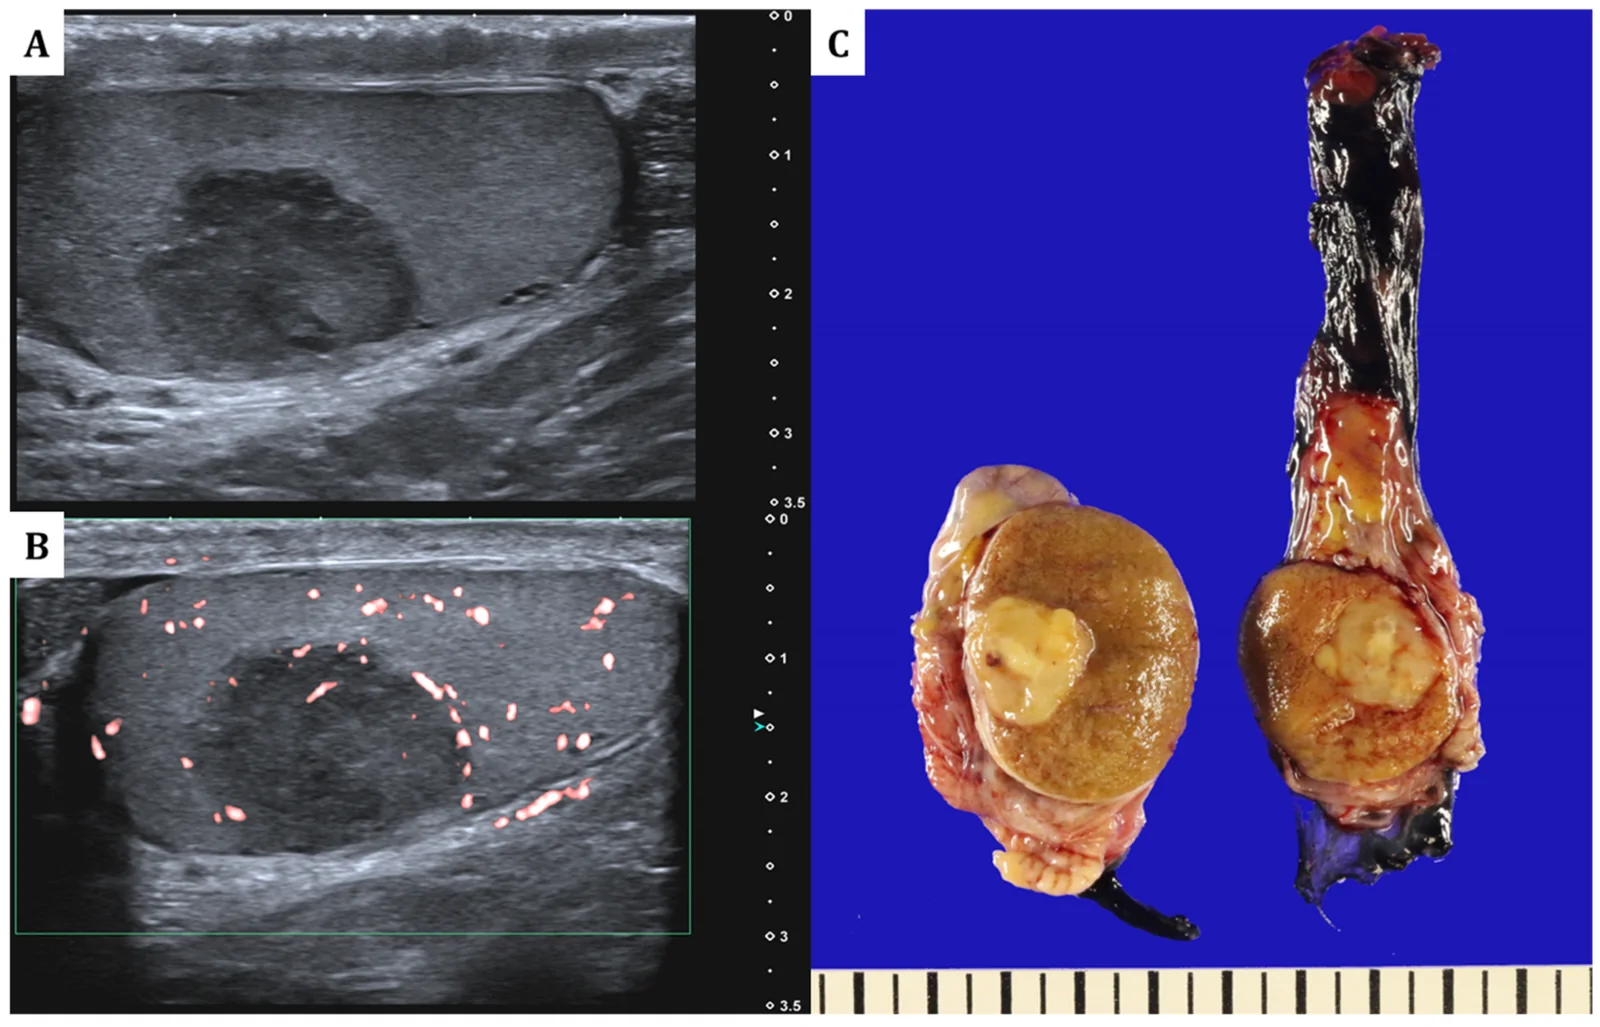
A 54-year-old man presented with a 3-month history of dull, intermittent pain in the left testis, rated 2/10 on the numeric rating scale. Serum tumor markers were within normal limits, including alpha-fetoprotein (AFP), 2.9 ng/mL (reference range, 0.56–7.56 ng/mL), and β-human chorionic gonadotropin (β-hCG), <0.2 mIU/mL. Gray-scale ultrasonography demonstrated a well-defined, heterogeneously hypoechoic intratesticular mass in the left testis (A). Superb microvascular imaging showed minimally increased internal vascularity (B). The patient subsequently underwent left radical inguinal orchiectomy. Gross examination revealed a well-circumscribed, tan-white intratesticular mass measuring 1.5 × 1.4 cm, without extratesticular extension (C; thick ruler markings indicate 1 cm intervals).
